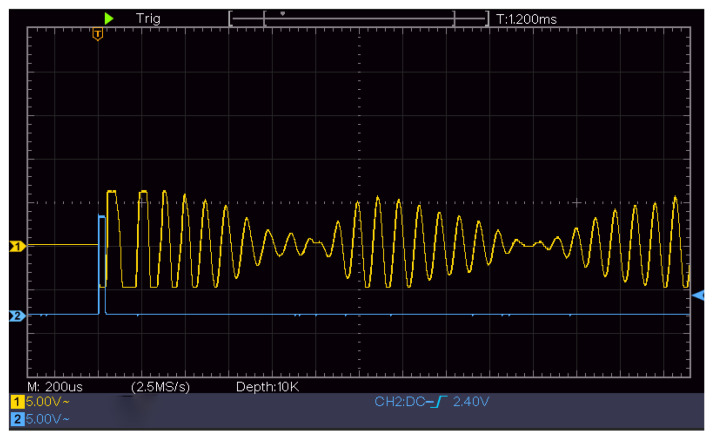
Negative half-wave representing first echo of ultrasonic transducer.
